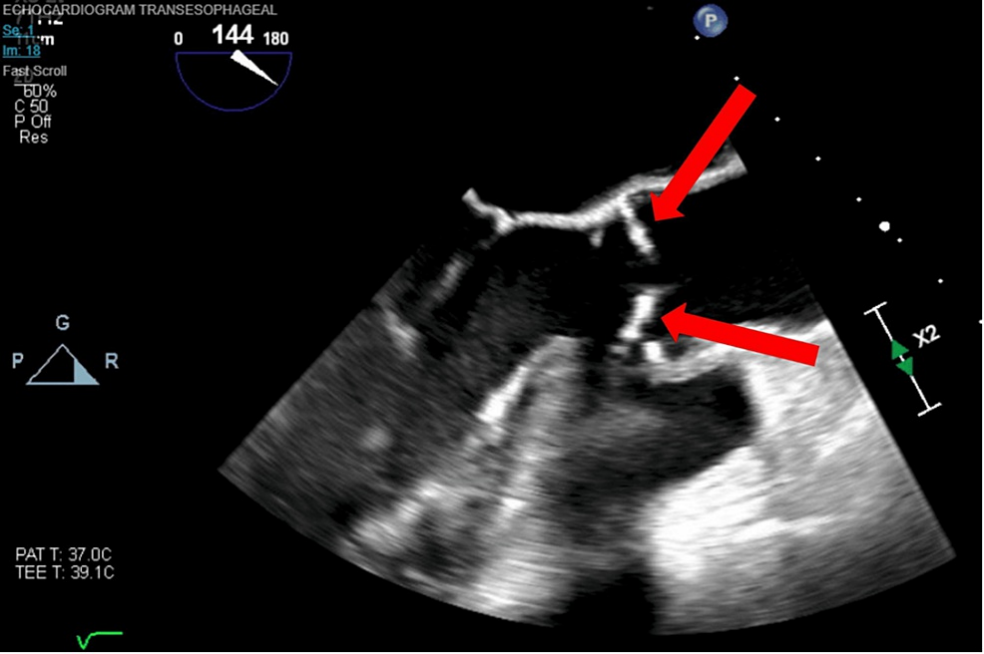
A transesophageal echocardiographic view of the aortic valve in the long axis showing thickened aortic valve leaflets with minimal opening leading to severe aortic stenosis (red arrows).
